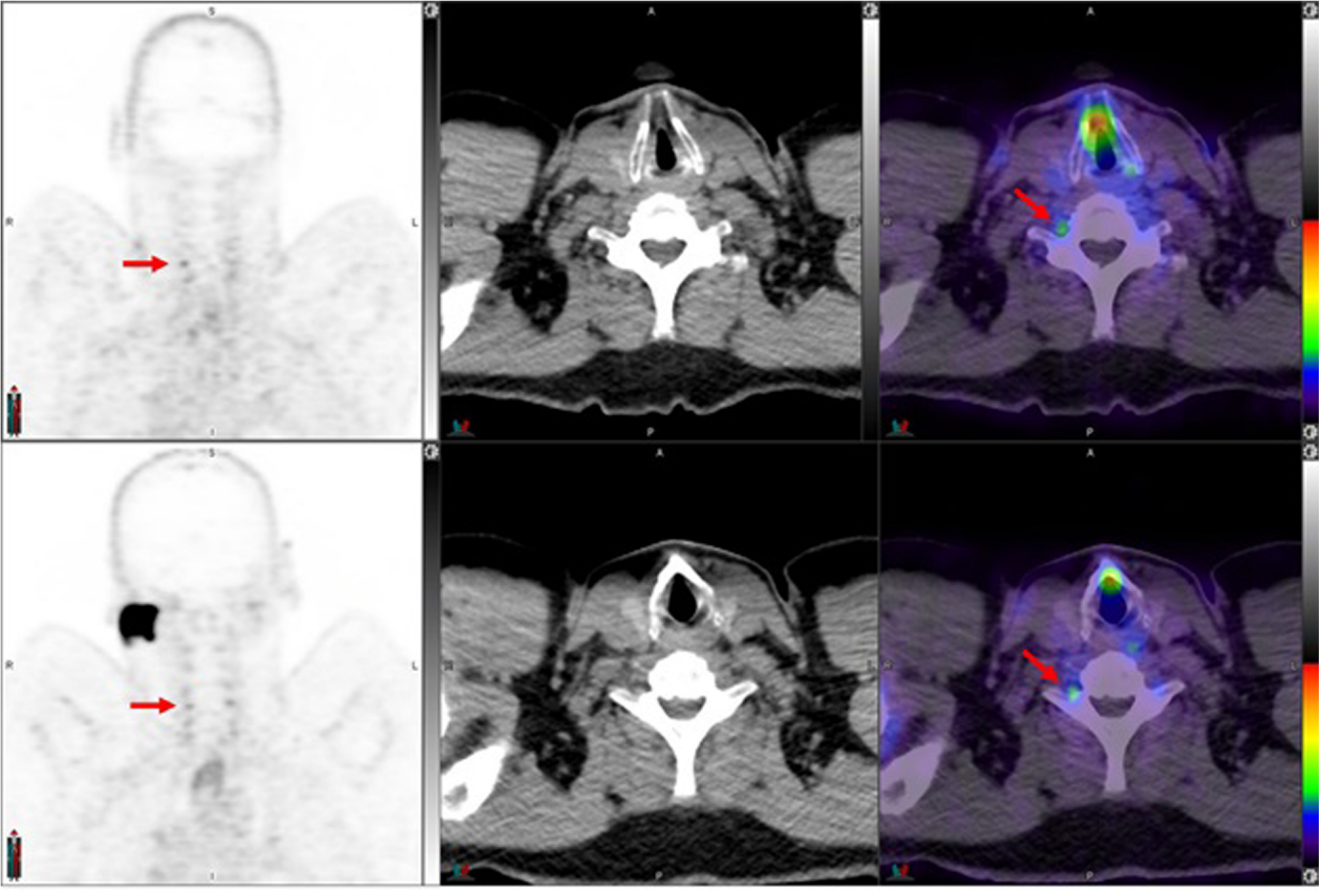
Top panel ^68^Ga-PSMA-11: coronal PET, axial CT and axial fused PET/CT. Right cervical ganglion (arrow) SUVmax = 2.4. Lower panel ^18^F –DCFPyL: PET, CT and PET/CT. Right cervical ganglion (arrow) SUVmax = 2.5. The color intensity has been slightly adjusted to highlight the uptake in the ganglia. Higher and bilateral uptake in lower cervical ganglia noted with ^18^F –DCFPyL
